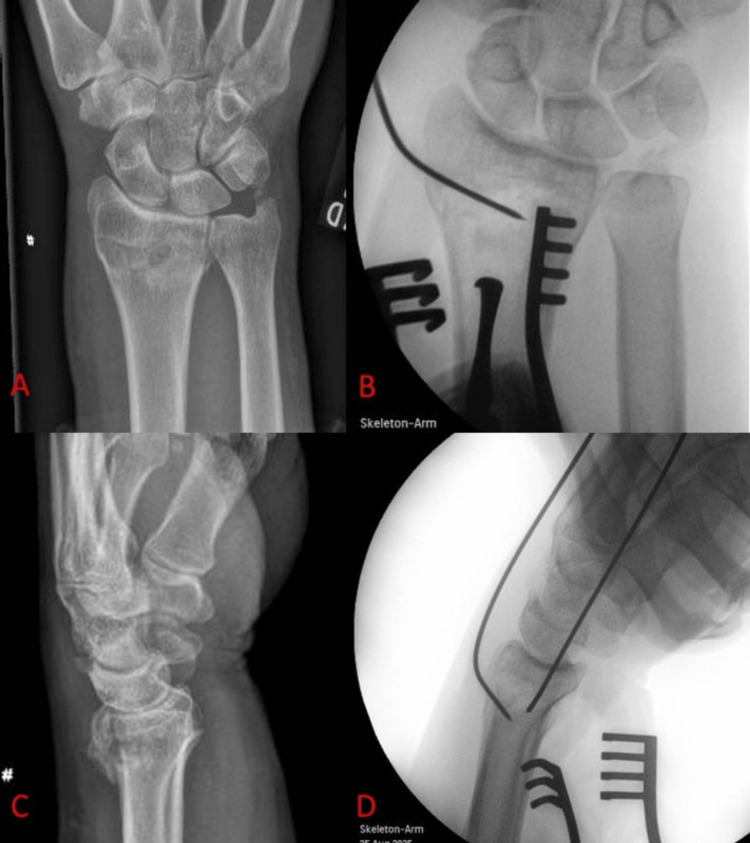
Radiographs demonstrating distal radius fracture reduction technique. (A) Anteroposterior radiograph of a distal radius fracture, demonstrating loss of radial height and inclination. (B) Intraoperative lateral radiograph showing a 1.6 mm wire correcting the coronal plane deformity. (C) Lateral radiograph of fracture, demonstrating dorsal tilt of distal fragment. (D) Intraoperative lateral radiograph showing second, dorsal 1.6 mm wire, resulting in complete fracture reduction.
